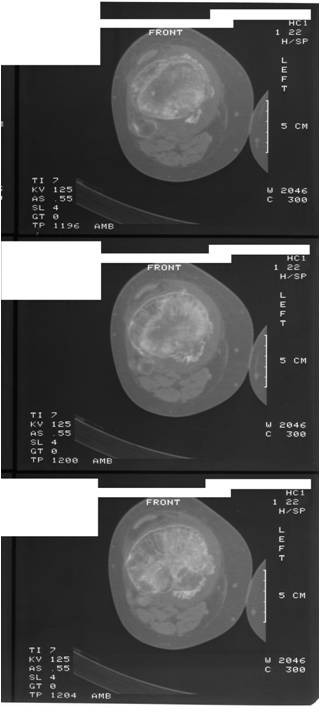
CT scan demonstrating the lesion in the proximal tibia.
